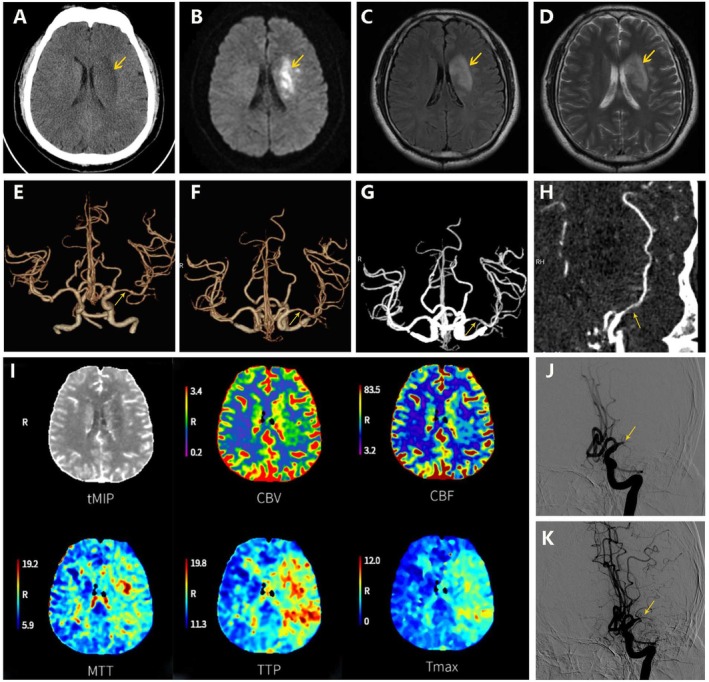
The patient received a series of examinations and was diagnosed as ischemic stroke of left basal ganglia region. (A) Head CT scan shows low density in the left basal ganglia region. (B–D) MRI demonstrated high DWI signal (B), slightly high Flair signal (C), and slightly elevated T2 signal (D) in the left basal ganglia region. (E–H) CTA demonstrated severe stenosis of the left middle cerebral artery (M1) from multiple angles, including 3D reconstruction (E, F), MIP (G), and MPA (H). (I) CTP revealed ischemic changes in the left cerebral hemisphere. (J) Two days later, DSA demonstrated occlusion at the origin of the left middle cerebral artery (J), with distal blood flow supplied via collateral circulation (K).
